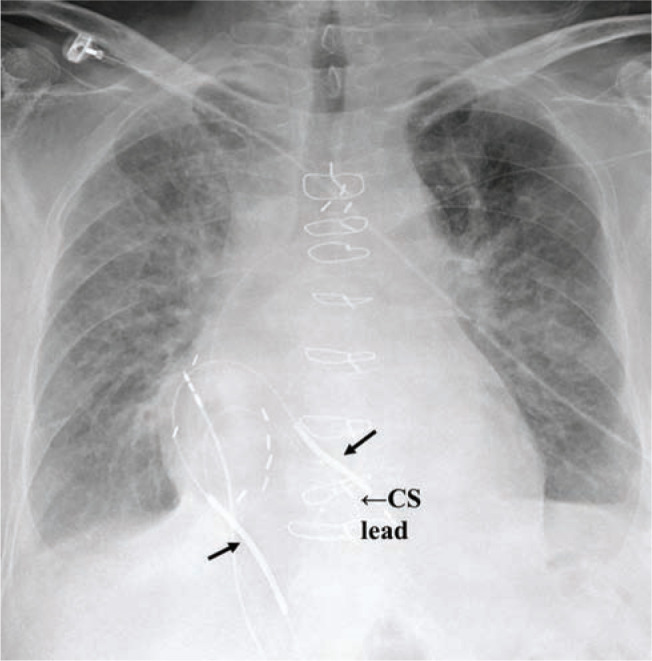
Postoperative AP chest radiography for Case 2 showing three intracardiac leads. The right atrial lead is along the lateral mid-RA, the LV CS lead is a proximal middle cardiac vein (labeled), and the RV lead in the lower septum with separation between the defibrillation coils (black arrows).
